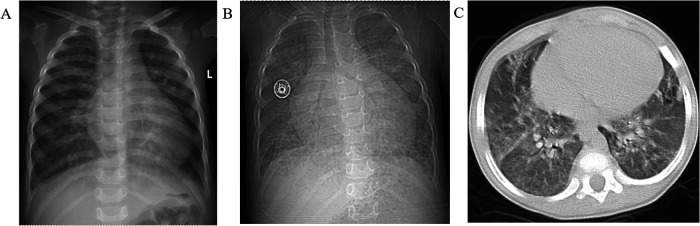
Chest radiographs showed progressive enlargement of the heart. (A) October 2023 results showed an enlarged heart, cardiothoracic ratio: 0.63, (B) December 2024 results showed further enlargement of the heart, cardiothoracic ratio: 0.69, (C) patient develops pulmonary edema.
